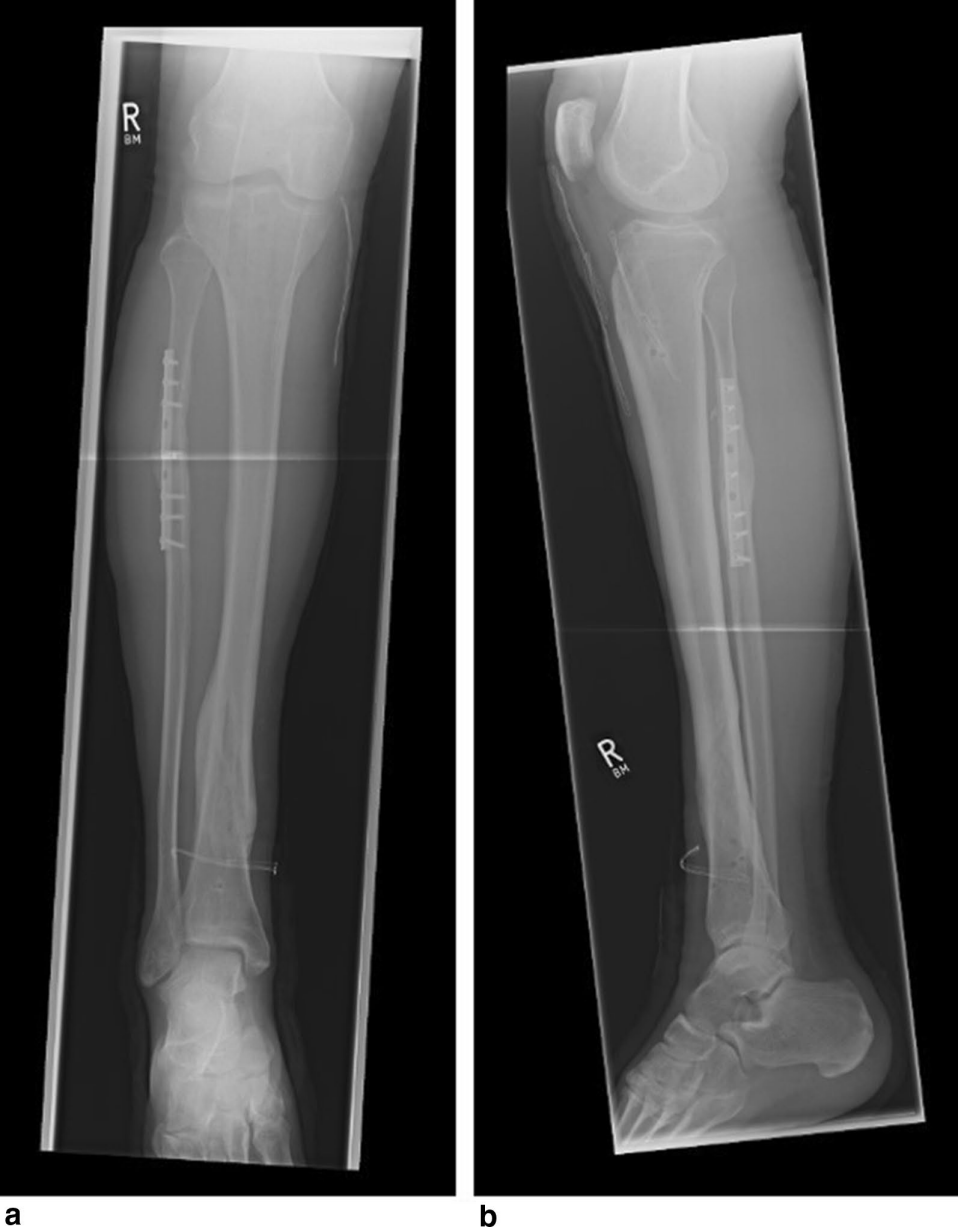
Anterio-posterior (a) and medio-lateral (b) radiographs 1 year after surgery, showing complete recovery of the tibial fracture and the remaining plate for fibula fracture reduction
